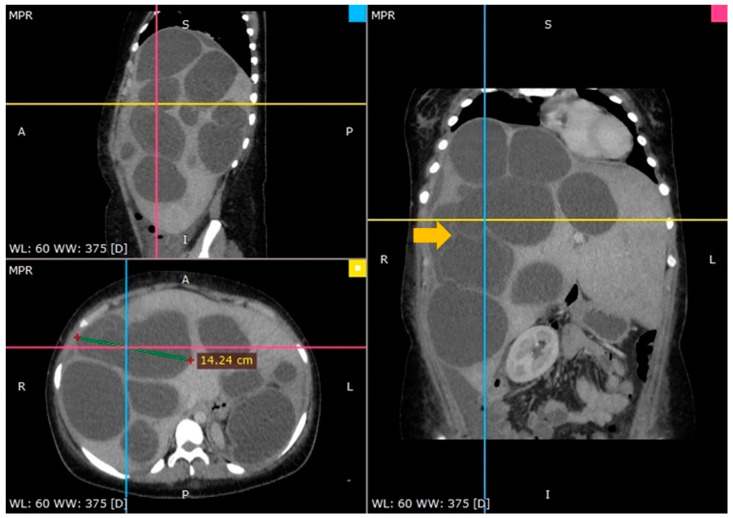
A CT scan showing multiple hydatid cysts in an enormously enlarged liver (arrow—floating internal membrane).
